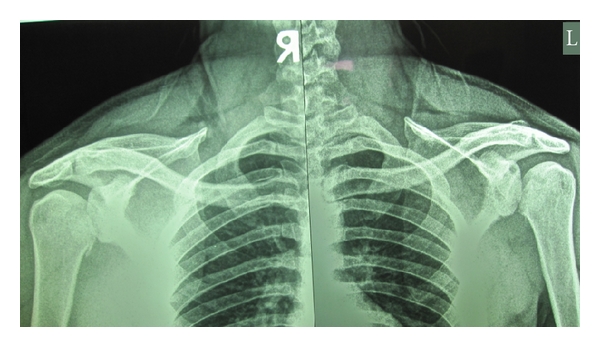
Anteroposterior X-ray shows bilateral posterior dislocation difficulty with absence of normal half-moon sign.
